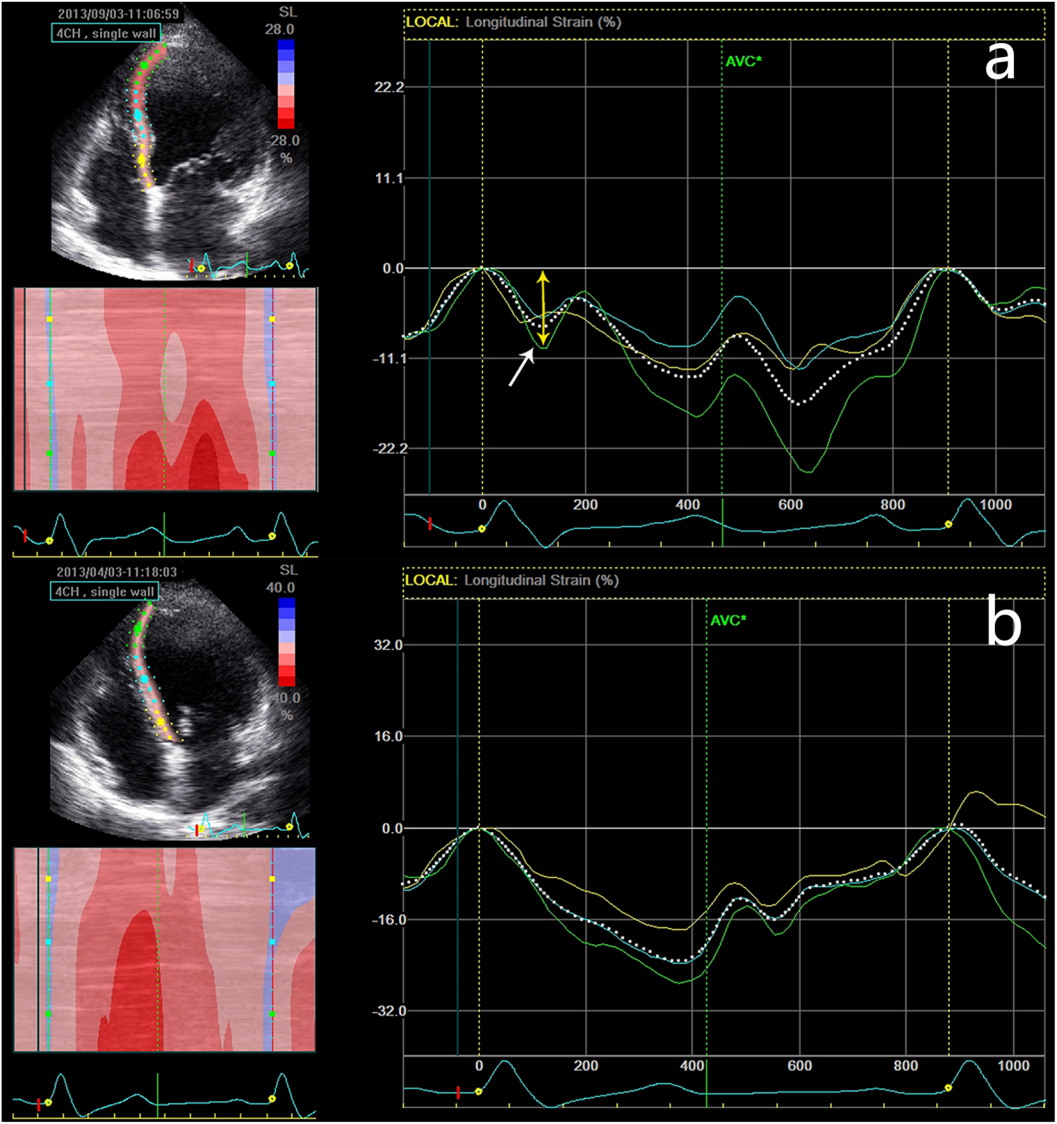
SF by 2D STE. Note the septal strain curve of LBBB-SF (a) and LBBB-NSF (b) patients. SF was defined as the presence of early leftward and then rightward septal motion within the isovolumic contractile period (white arrow). The magnitude of SF was defined as the maximal early negative peak strain in the involved septal myocardial segments (yellow arrow). LBBB, left bundle branch block; SF, septal flash; 2D STE, two-dimensional speckle-tracking echocardiography.
